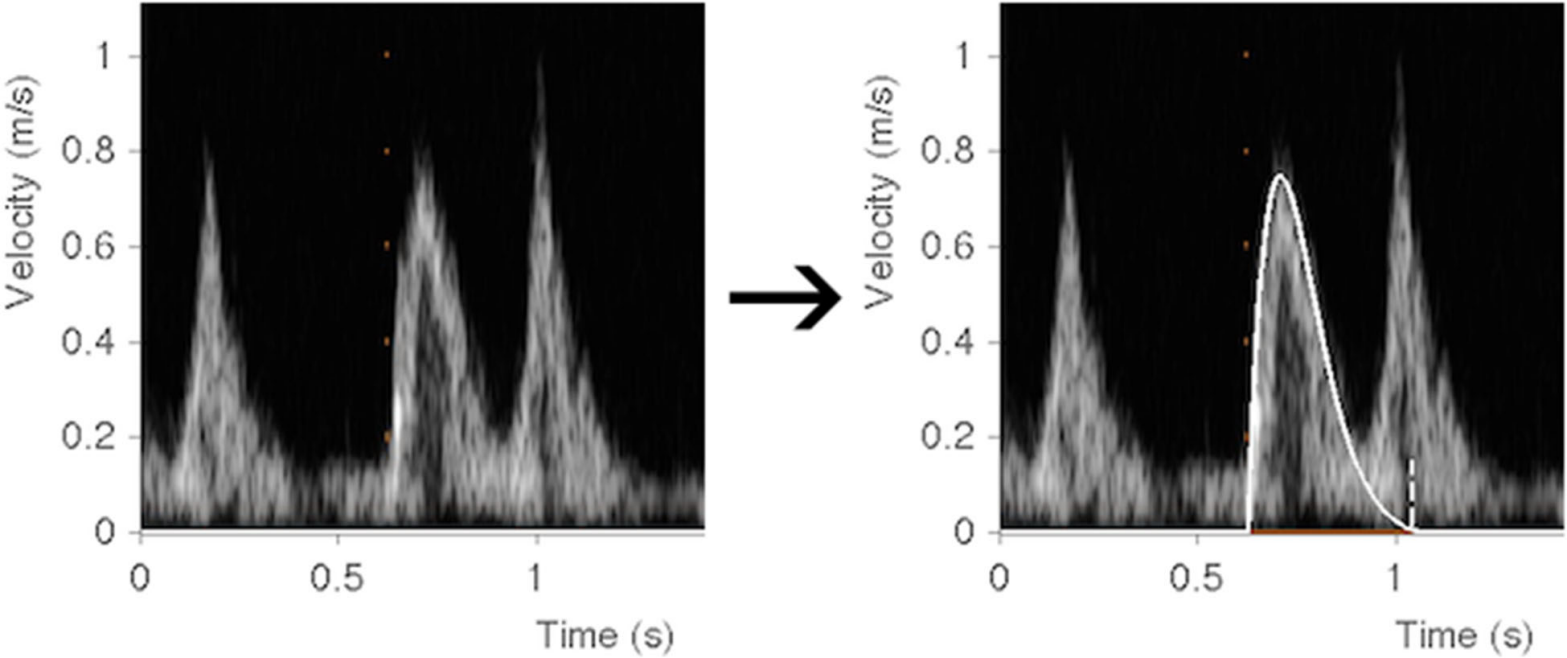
The figure shows an E-wave from pulsed-wave Doppler measurement of the flow across the mitral valve. The solid white line (right panel) shows the curve fit of the PDF method whereby the mathematical formula describing a damped harmonic oscillator is fit to the contour of the E-wave, thus generated the PDF measures. Analysis was performed using the freely available software www.echoewaves.org
